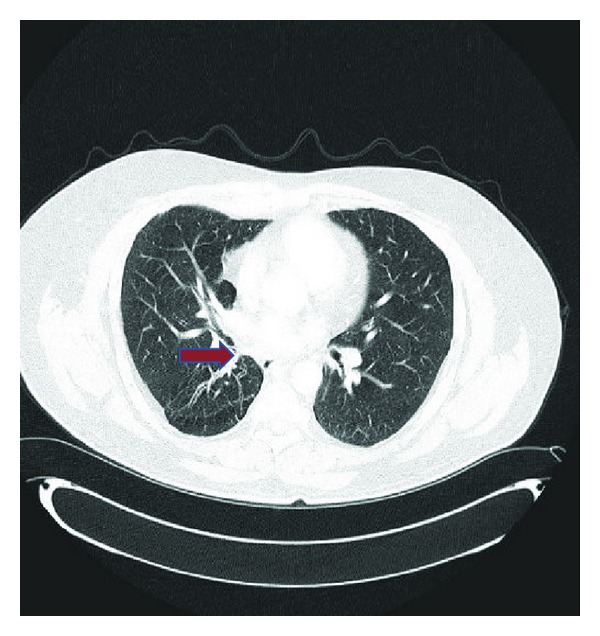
CT scan showing endobronchial mass (red arrow).
